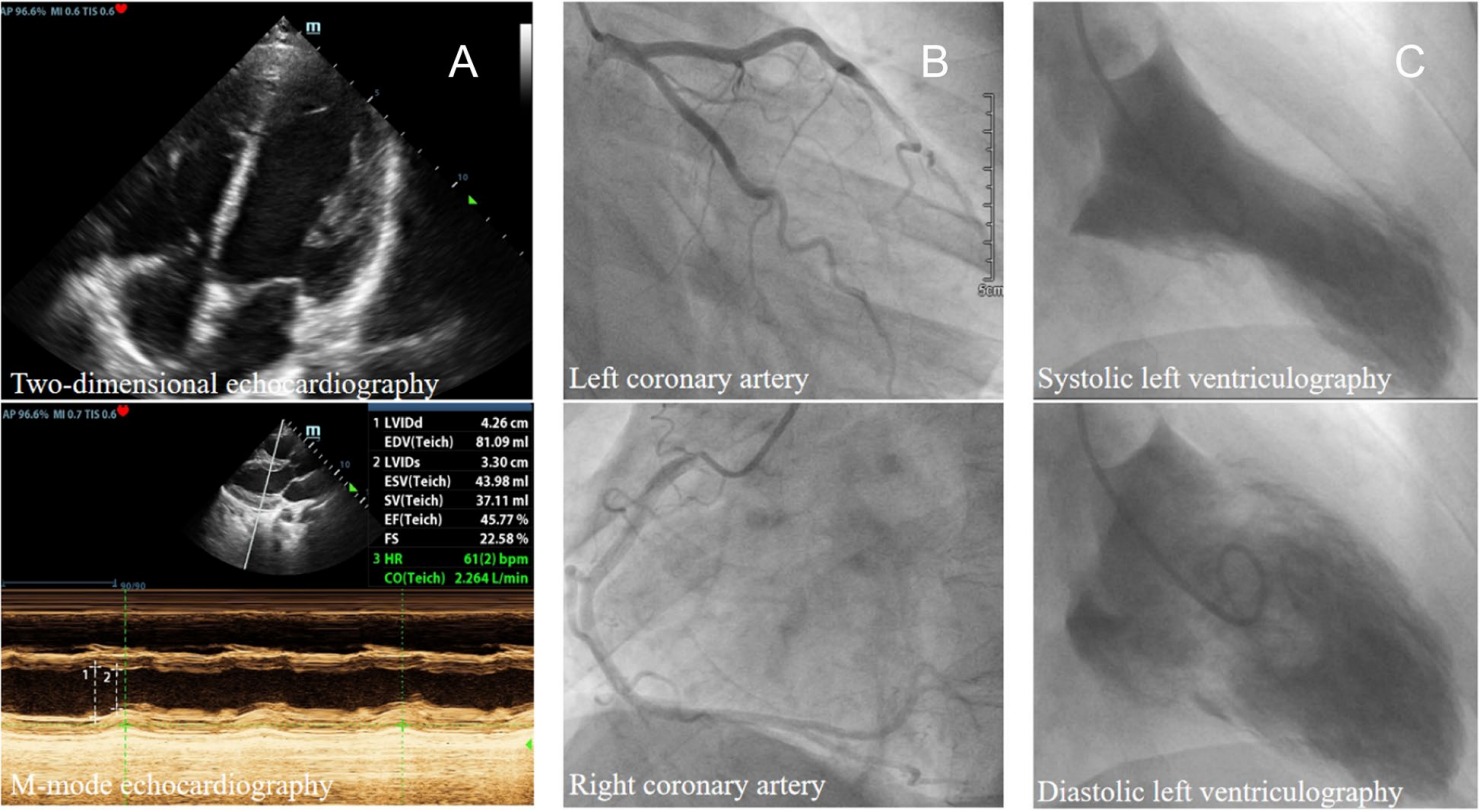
Two-dimensional and M-mode echocardiogram on the third postoperative day (A); left and right coronary angiography (B); revealed a new left ventricular apex akinesis and apical ballooning during systole (C)
